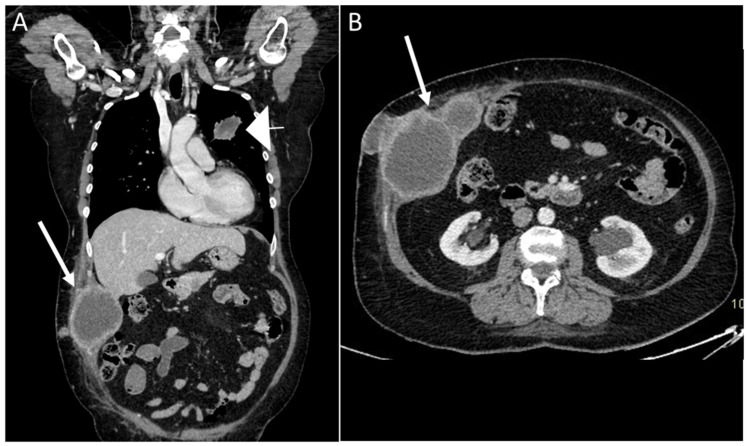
A 68-year-old woman with a palpable solid mass on the abdominal wall. Coronal and axial CT scan on portal venous phase showed the presence of a primary lung cancer tumor (A), arrowhead) with multiple implants, including the abdominal wall (arrows, A and B).
